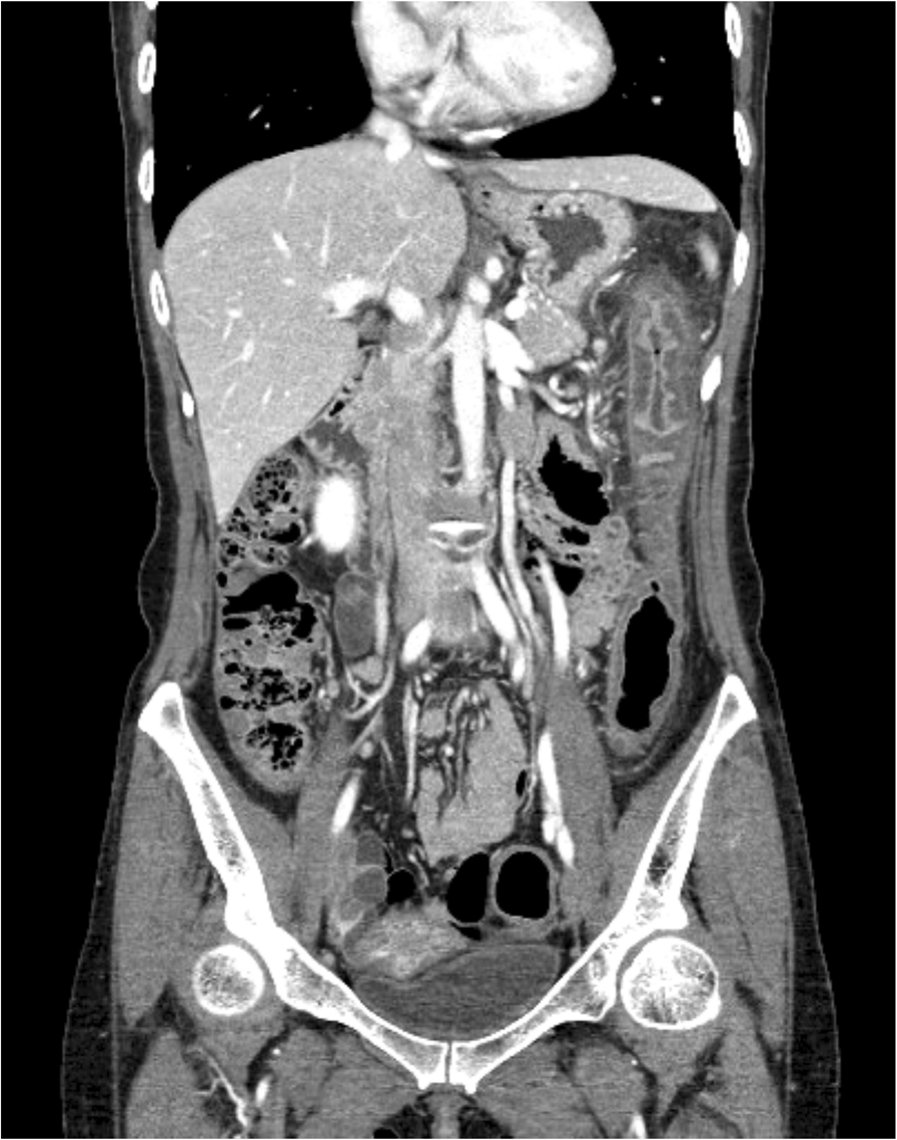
Computed tomography demonstrating segmental edematous wall thickening of the descending and sigmoid colon
